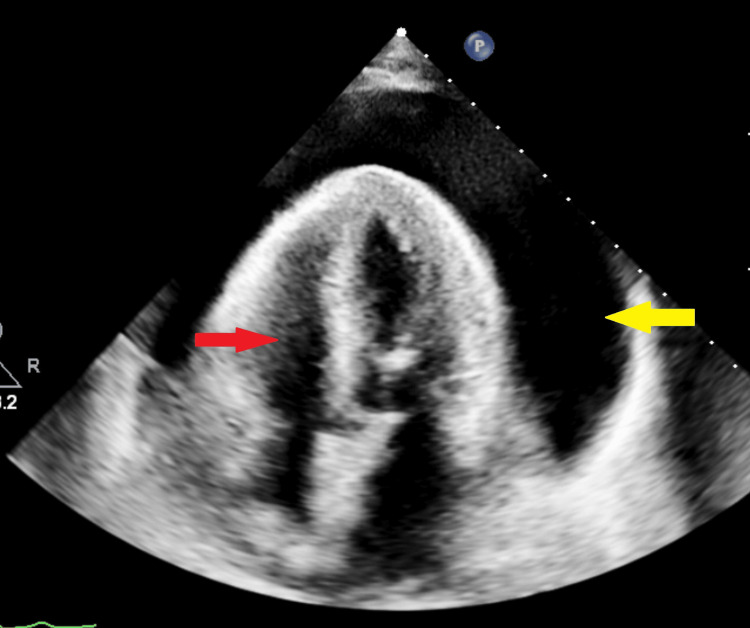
Echocardiogram showing apical four chamber view with large circumferential pericardial effusion (yellow arrow) and right ventricle (red arrow) for reference
